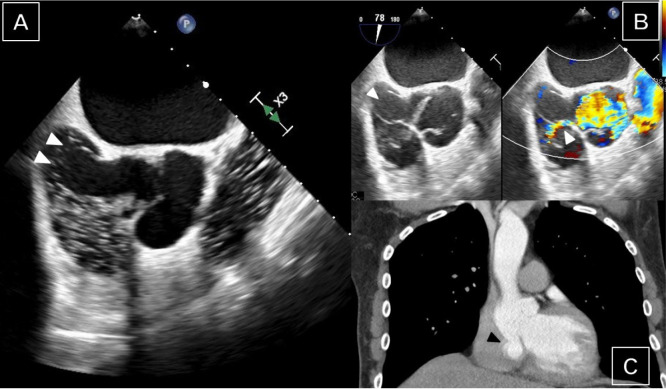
Preoperative transesophageal echocardiography (A, B) and contrast-enhanced
